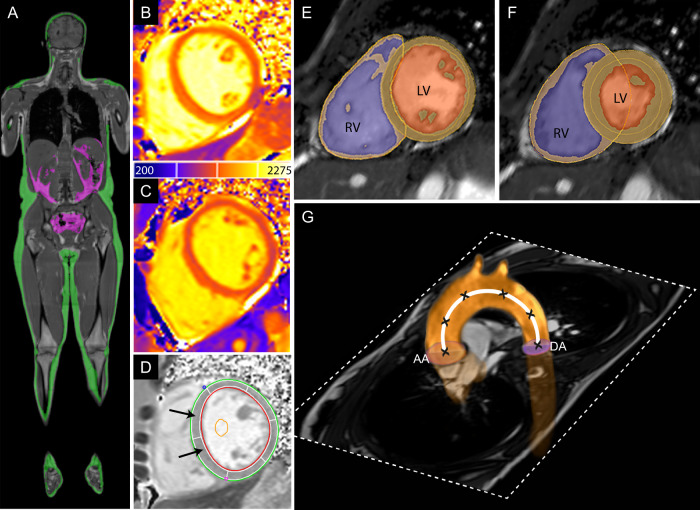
Magnetic resonance images demonstrating the assessment of cardiac function and mass, native T1, pulse-wave velocity, and whole-body fat. A Whole body fat. Visceral (green) and subcutaneous (purple) adipose tissue segmentations shown on coronal reformat of whole-body fat image. B–D Native T1. Representative native T1 map for healthy control (B) and a patient with chronic schizophrenia (C). Each slice was semi-automatically segmented with endocardial (red) and epicardial (green) borders and divided into six segments (D). Native myocardial T1 time was calculated as the mean T1 time the two septal segments (black arrows). E, F Ventricular function and mass. Endocardial and epicardial contours of the left and right ventricle in diastole (E) and systole (F). Segmentation shows myocardium (orange), left ventricular (LV) cavity (dark orange), and right ventricular (RV) cavity (purple). G Pulse-wave velocity. Transverse cardiac magnetic resonance imaging slice through the aortic arch displaying the magnitude (dotted white slice) with velocity encoded flow through the ascending aorta (AA) and descending aorta (DA). Path length was determined by markers (X) creating a three-dimensional Bezier curve (white line) through the centre of the aorta that intersected the plane at which flow measurements were acquired.
